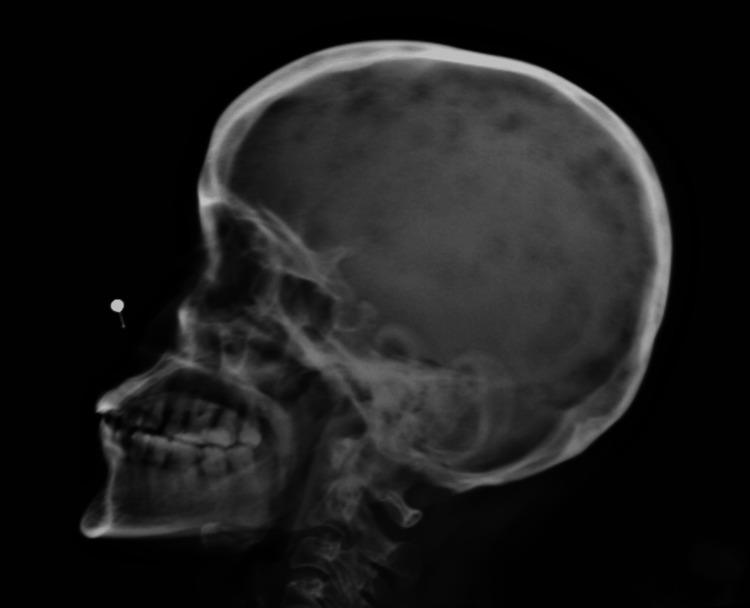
Lateral skull X-ray demonstrating multiple lytic lesions in marginal zone lymphoma Lateral skull radiograph of the present case shows multiple well-defined, punched-out lytic lesions without surrounding sclerosis, indicative of extensive osteolytic bone involvement. The absence of periosteal reaction or reactive bone formation suggests a hematologic malignancy-driven osteolysis.
